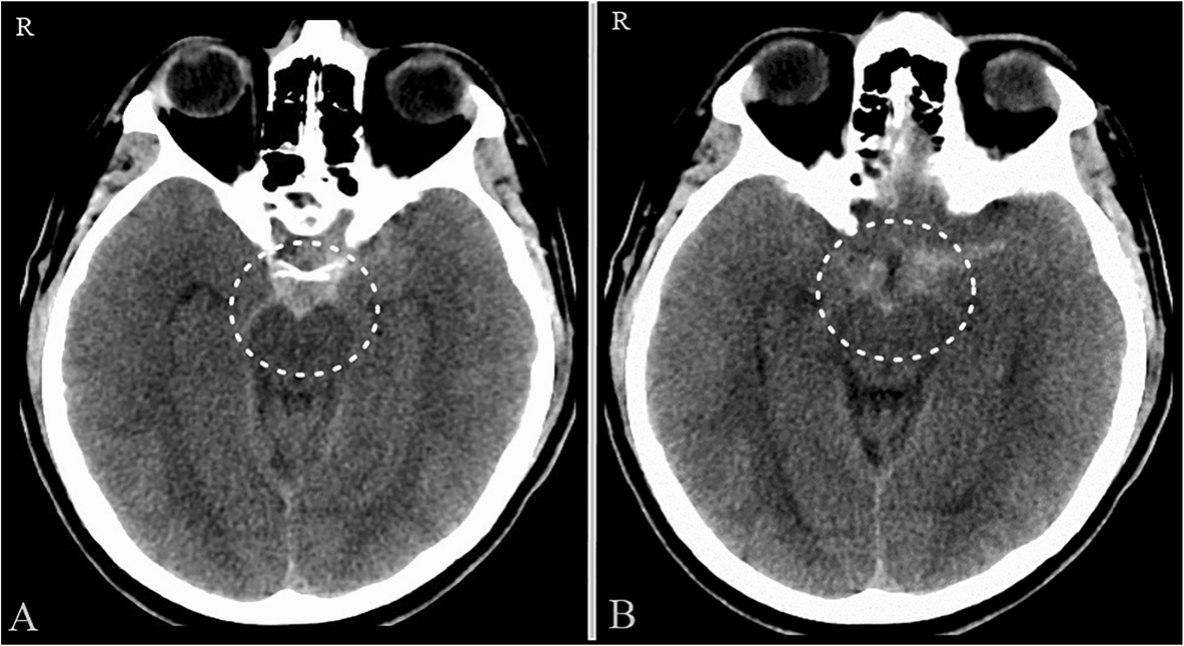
Case 2. Axial non-contrast computed tomography of the head (A and B) shows perimesencephalic spontaneous subarachnoid hemorrhage (circle) with the center of bleeding located anterior to the midbrain.
